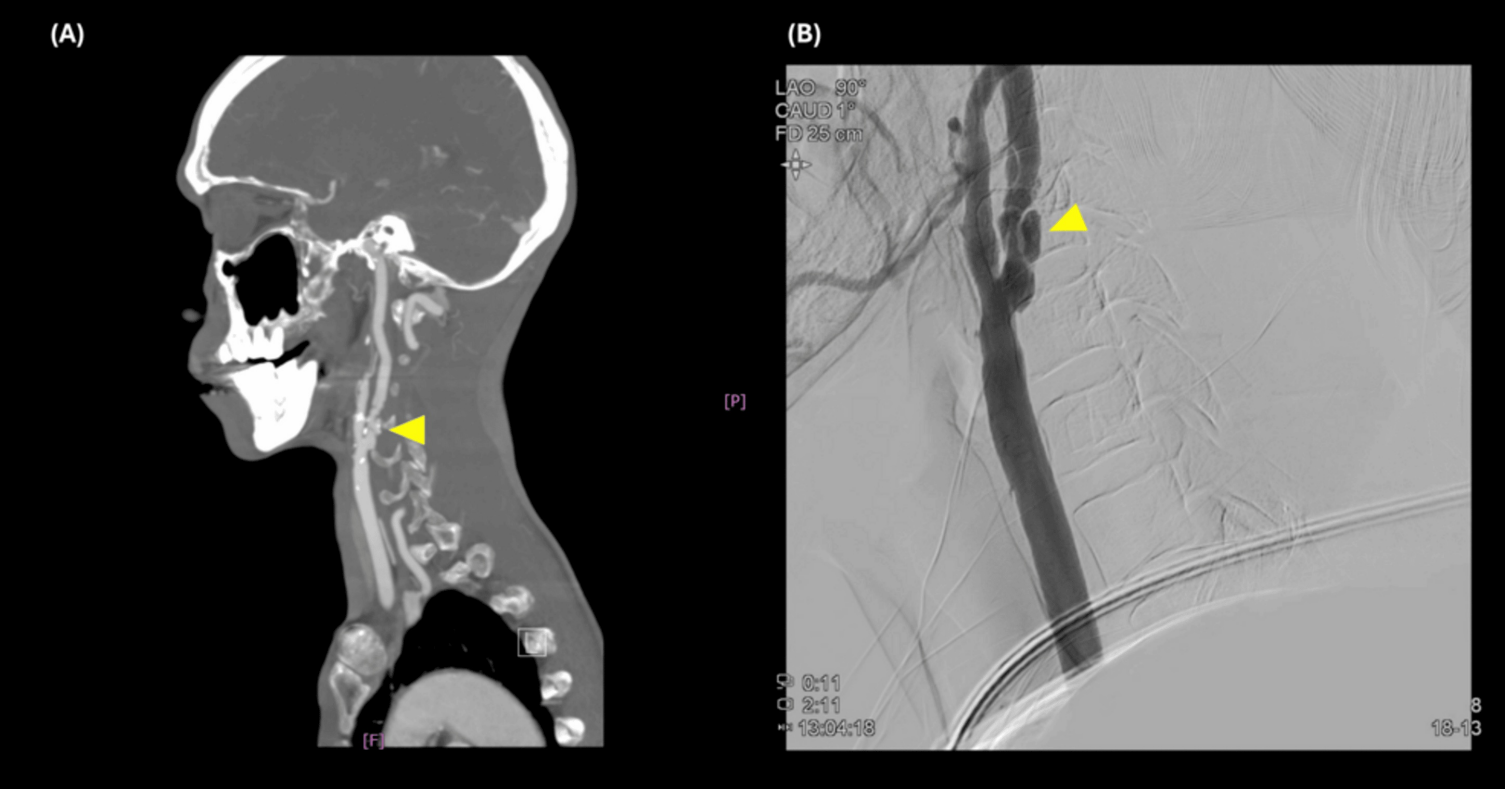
CTA (A) and DSA (B) showing high-grade stenosis (arrowhead) of the left proximal cervical ICA CTA: computed tomography angiography; DSA: digital subtraction angiography; ICA: internal carotid artery
